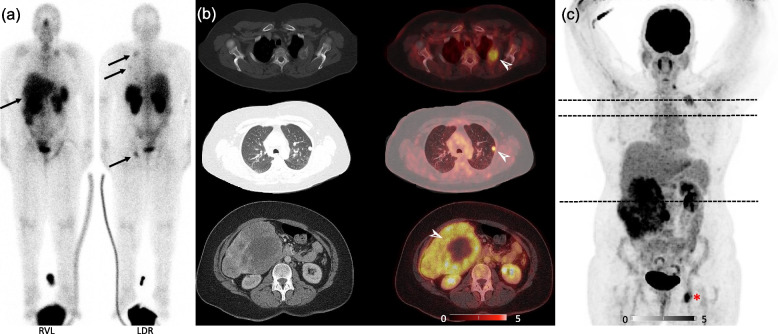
a Post-therapeutic whole-body scintigraphy after administration of 7.4 GBq Lu-177-DOTATOC showing suspicious radiotracer accumulation in lesions of liver, left lung and bone (black arrows). (b) Axial slices of CT and fused images with 18F-FDG PET of thorax and abdomen. Three tracer accumulations suspected of metastasis (osseous, pulmonary and hepatic) are highlighted (white arrowhead). c Maximum intensity projection (MIP) of 18F-FDG PET/CT. The dashed line marks the exemplary axial slices in B. Additional hypermetabolic lesions can be found for example in left iliac bone (red asterisk). *Abbreviations:* LDR = left-dorsal-right projection; RVL = right-ventral-left projection
